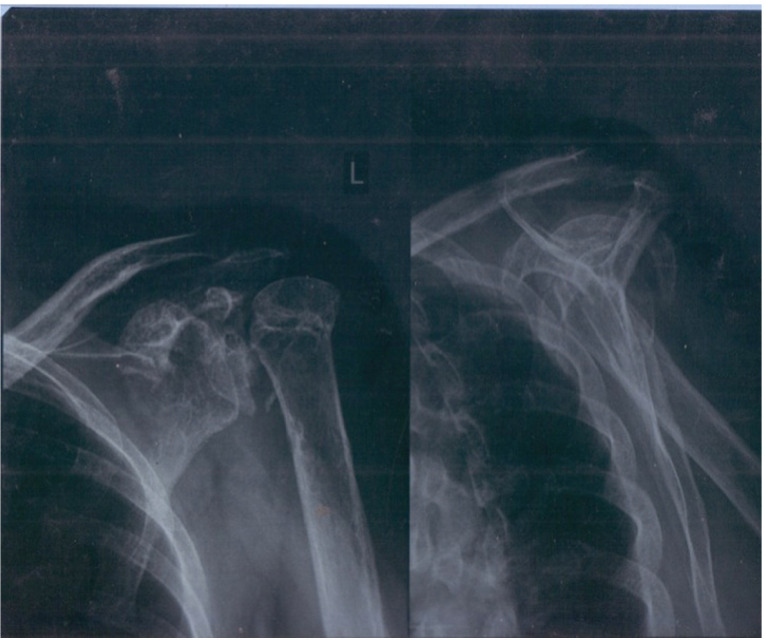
X-ray in ER department; Abbreviation: L—left.
